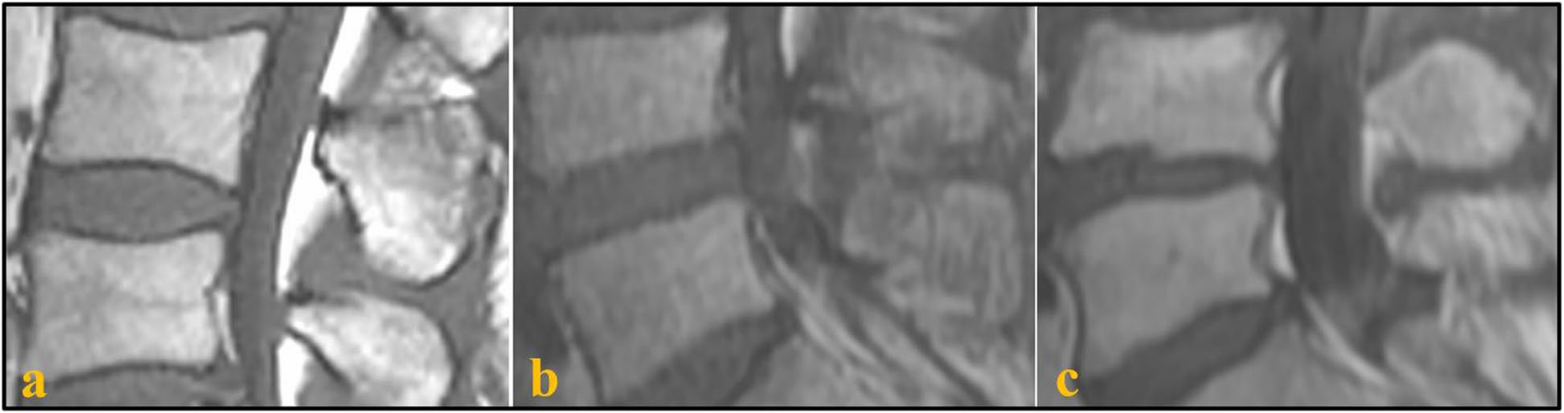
The classification of the disk space morphology on MRI. a concave. b flat. c irregular
